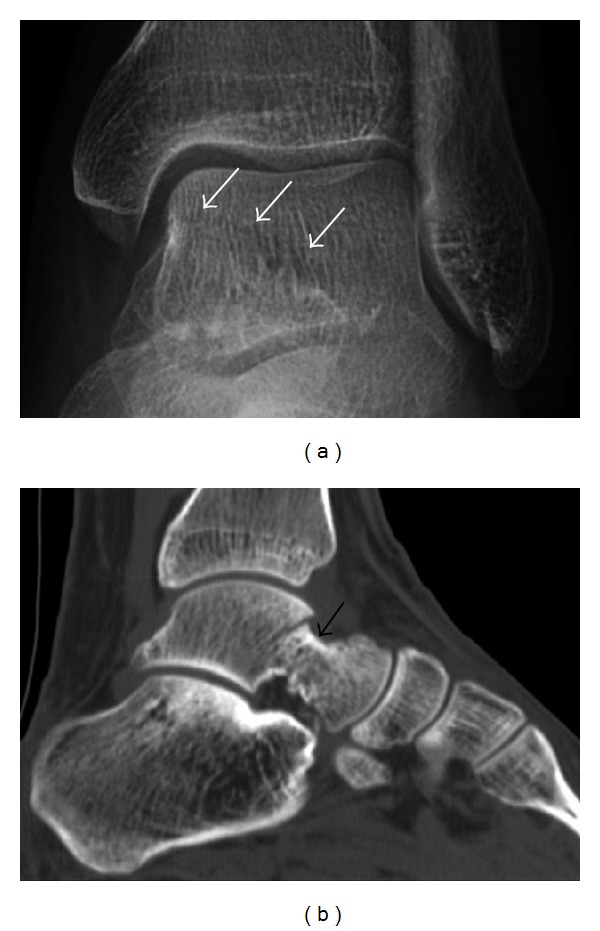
Subtle anterior talar fracture in a 39-year-old man presenting with ankle pain after a fall. (a) Anteroposterior radiograph shows a subtle oblique radiolucent line through the talus (white arrows). (b) Sagittal CT reformation confirms the presence of an anterior talar fracture with cortical offset (black arrow).
